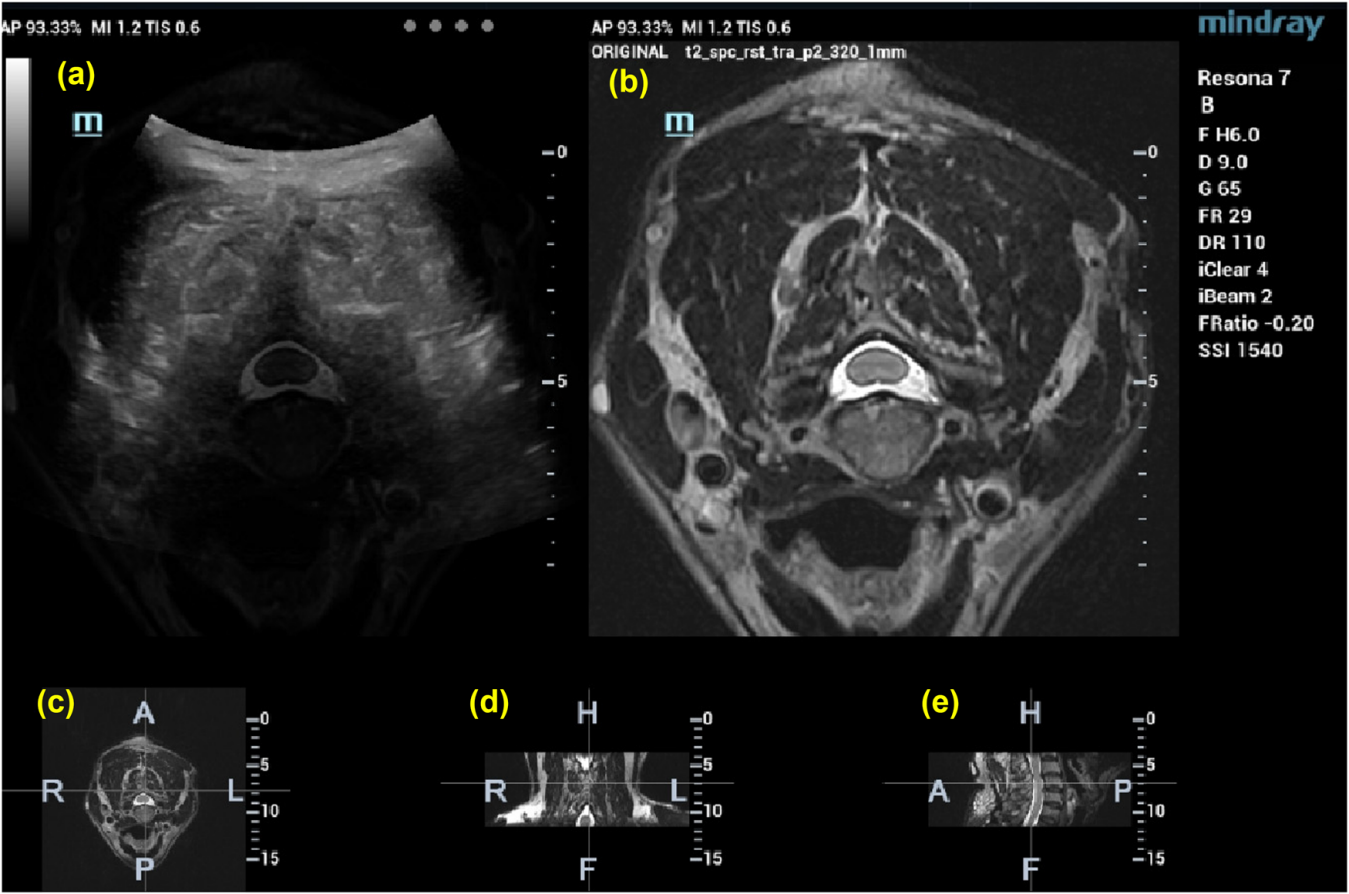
Images of cervical vertebrae after US-MRI fusion: (a) US-MRI fusion images, (b) MRI images, (c) cross section, (d) coronal section, and (e) sagittal section.
